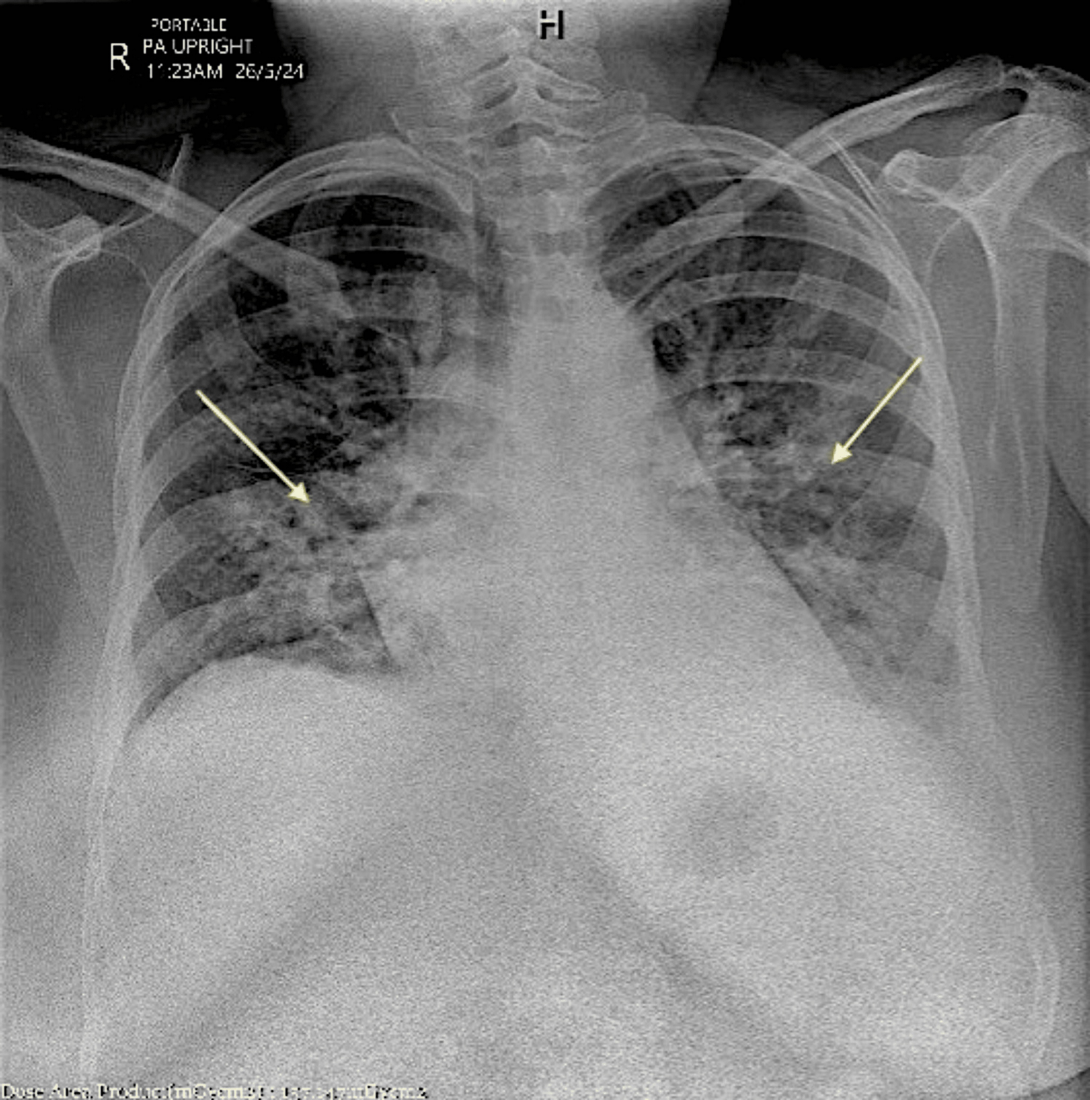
Chest X-ray Right-sided interstitial consolidation in the right lower lobe, with possible early interstitial consolidation at the base of the left lower lobe. Additionally, bilateral increased interstitial markings and perihilar bronchial wall thickening were observed, indicating infection; however, no pulmonary congestion was noted. The heart size and mediastinum were normal, and both hilar regions appeared unremarkable, showing no evidence of pneumothorax. (Yellow arrows are added to indicate the right-sided interstitial consolidation in the right lower lobe and likely early interstitial consolidation at the base of the left lower lobe, reflecting the presence of infection. The overall lung fields demonstrate bilateral increased interstitial markings, consistent with infection, while no pulmonary congestion or pneumothorax is observed.)
